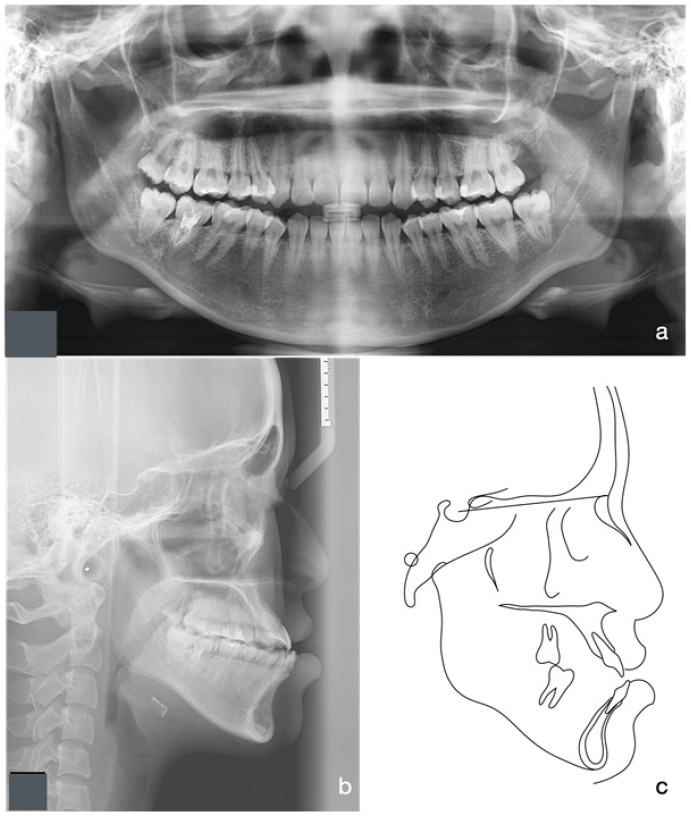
Initial Orthopantomographic (OPG) and lateral cephalometric radiographs and tracing. Orthopantomographic (OPG) radiographic examination revealed no significant caries or pathological conditions, and the patient exhibited good periodontal status. The lower right second molar had undergone endodontic treatment with no apical lesion present (a). Cephalometric radiograph and tracing showed a skeletal Class III relationship with an average Frankfort-mandibular plane angle (b,c).
